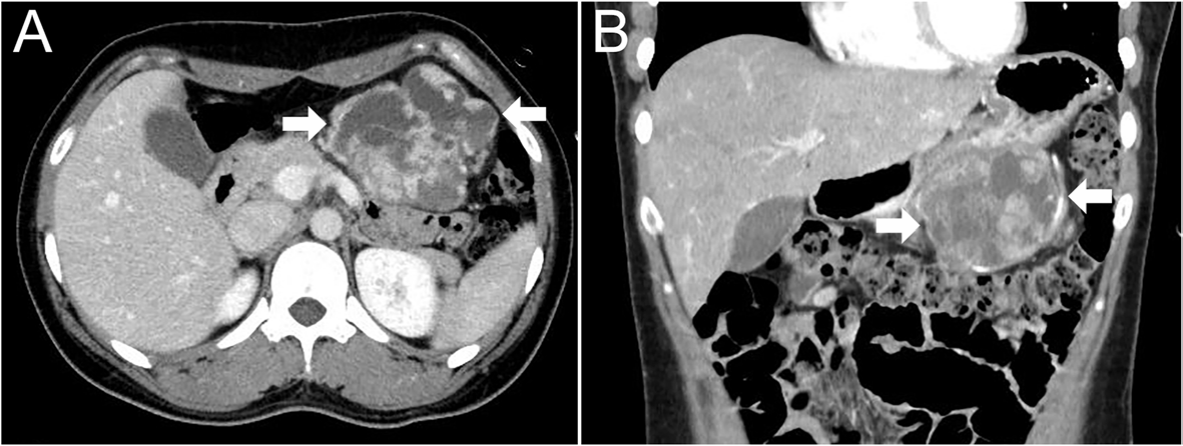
A 29-year-old Japanese woman with gastroblastoma. A Axial plane enhanced computed tomography (CT) image of the abdomen. The gastroblastoma appears as a well-circumscribed mass (arrows) showing a multi-cystic, heterogeneously enhanced mass in the lower part of the stomach; B Coronal plane enhanced CT image of the abdomen. Multi-cystic nature and heterogeneity of the gastroblastoma (arrows) arising from the bottom of the gastric wall in the upper-left quadrant
